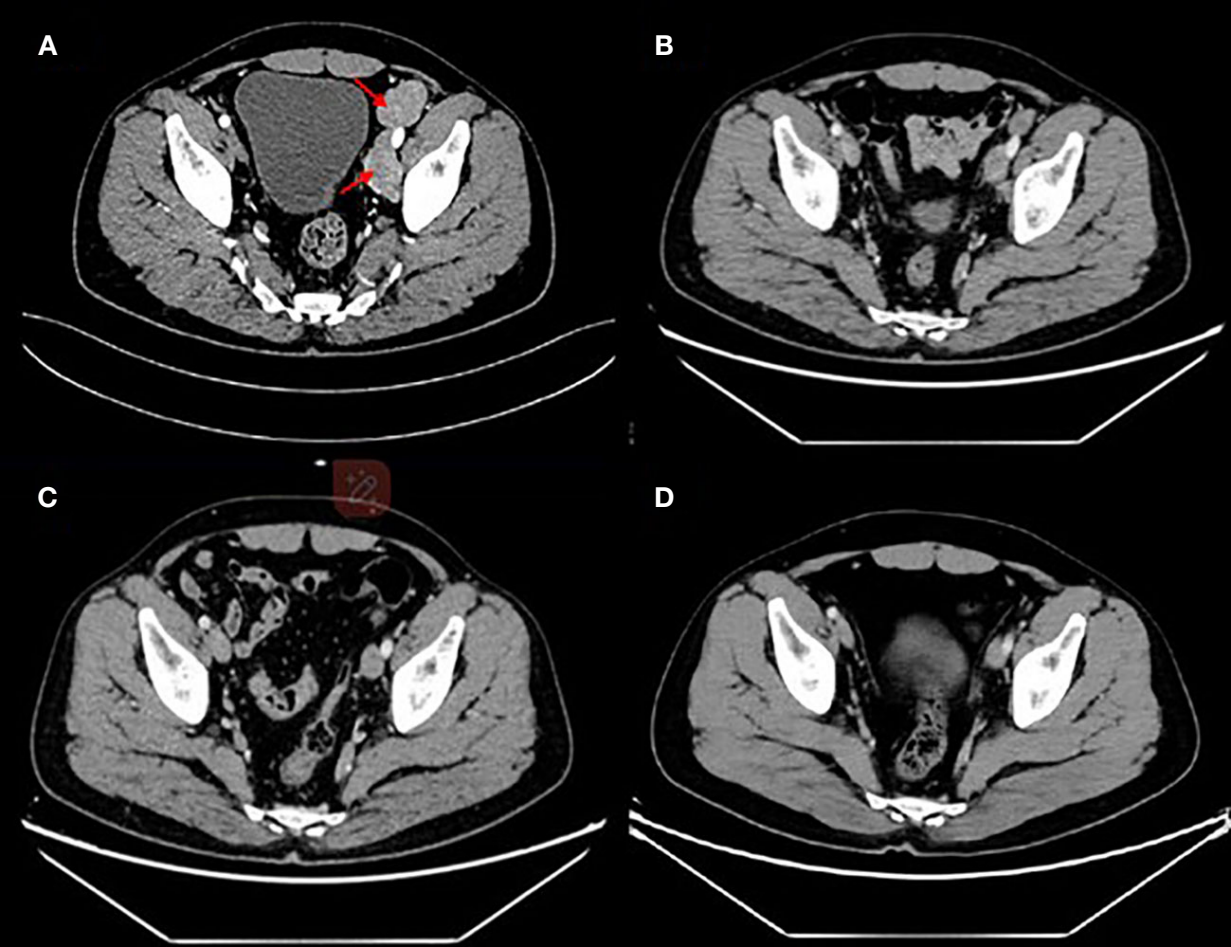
Enhanced computed tomography for efficacy evaluation (A) before treatment on 29 June 2022, (B) after two cycles of disitamab vedotin plus serplulimab on 7 September 2022, (C) after five cycles of disitamab vedotin maintenance therapy on 27 July 2023, and (D) 9 months after stopping any treatments on 24 March 2024.
